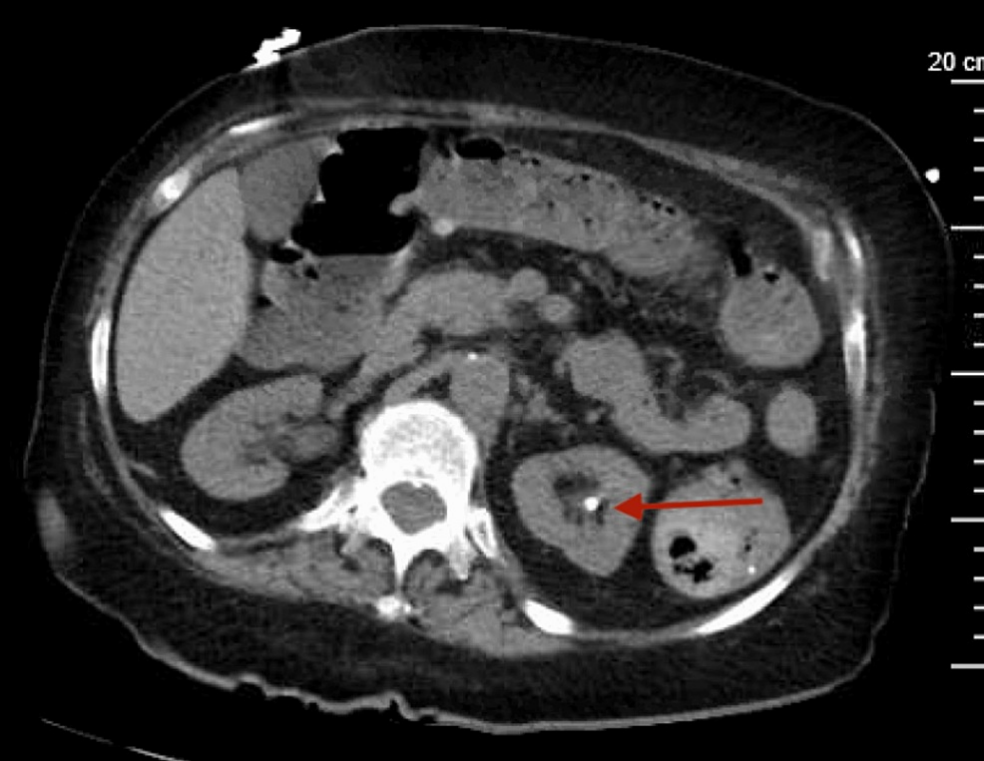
CT abdomen showing 4.7-mm non-obstructing calcification in the left kidney (red arrow)
